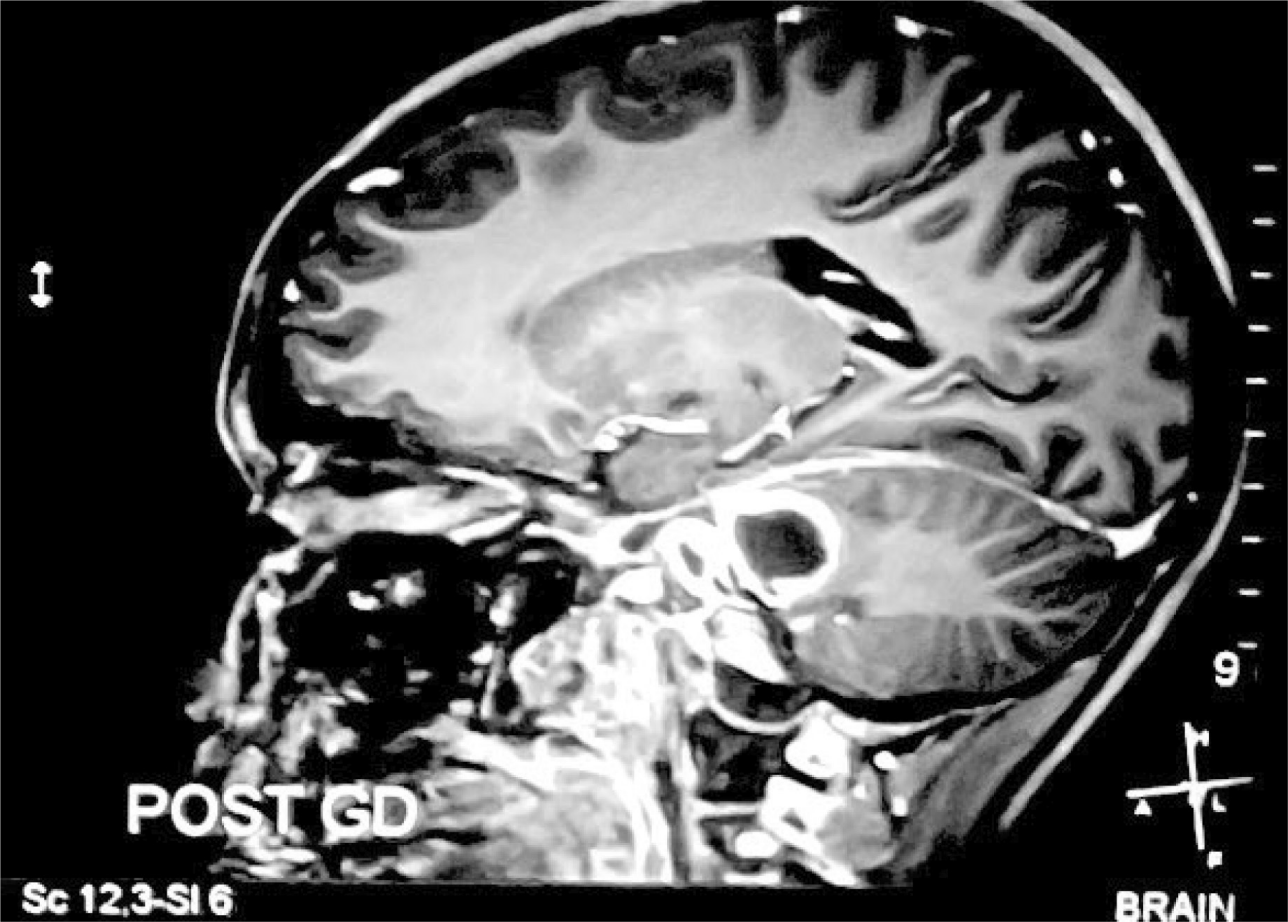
Sagittal view of contrast‐enhanced T1 weighted MRI of head showing rim‐enhancing cystic lesion in left pons and within the left side of the cerebellopontine angle.
